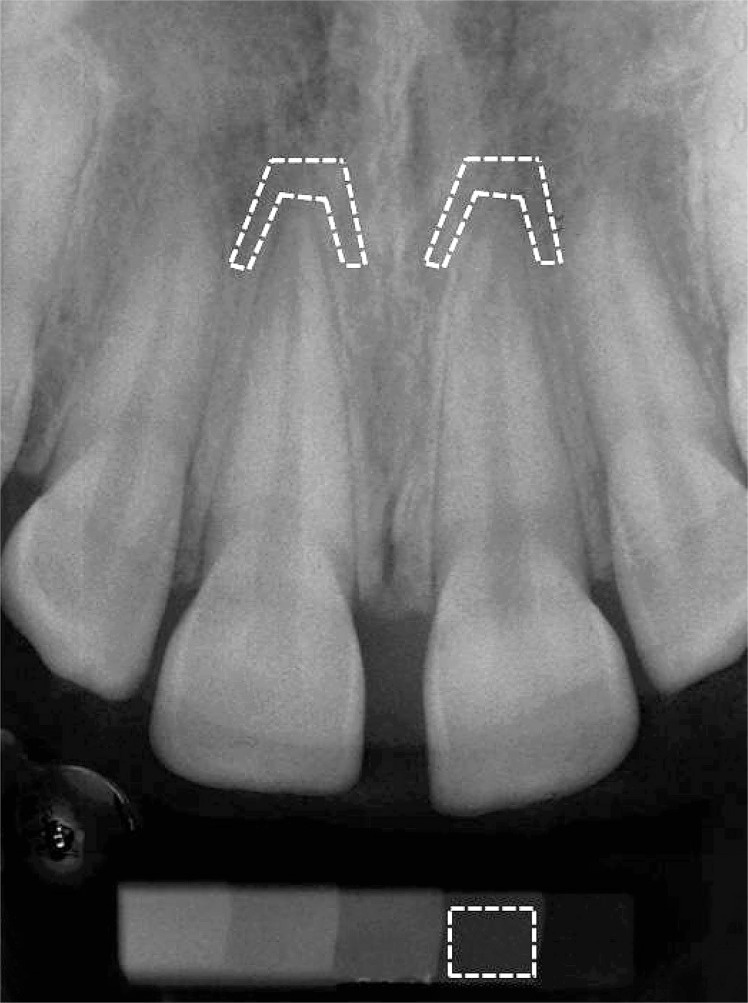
Scanned periapical radiograph; region of interest selected in the apical
